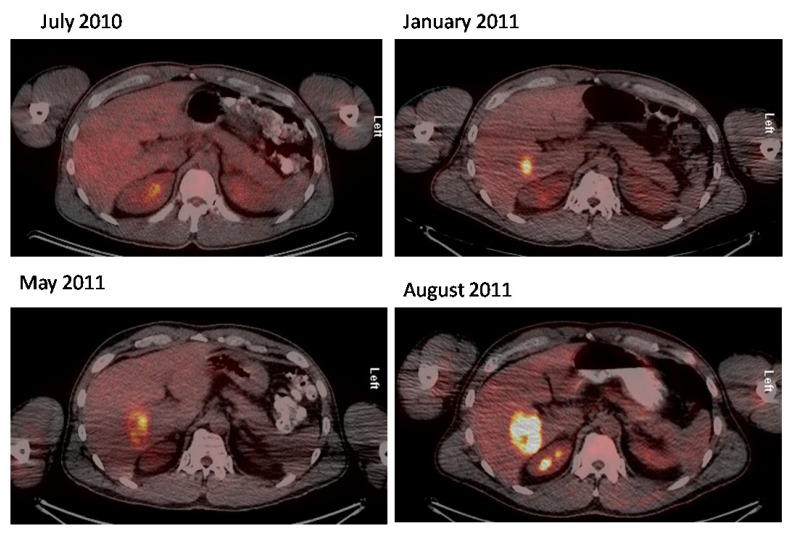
A series of axial fused PET/CT images showing gradual progress in liver metastasis from Merkel cell carcinoma.
